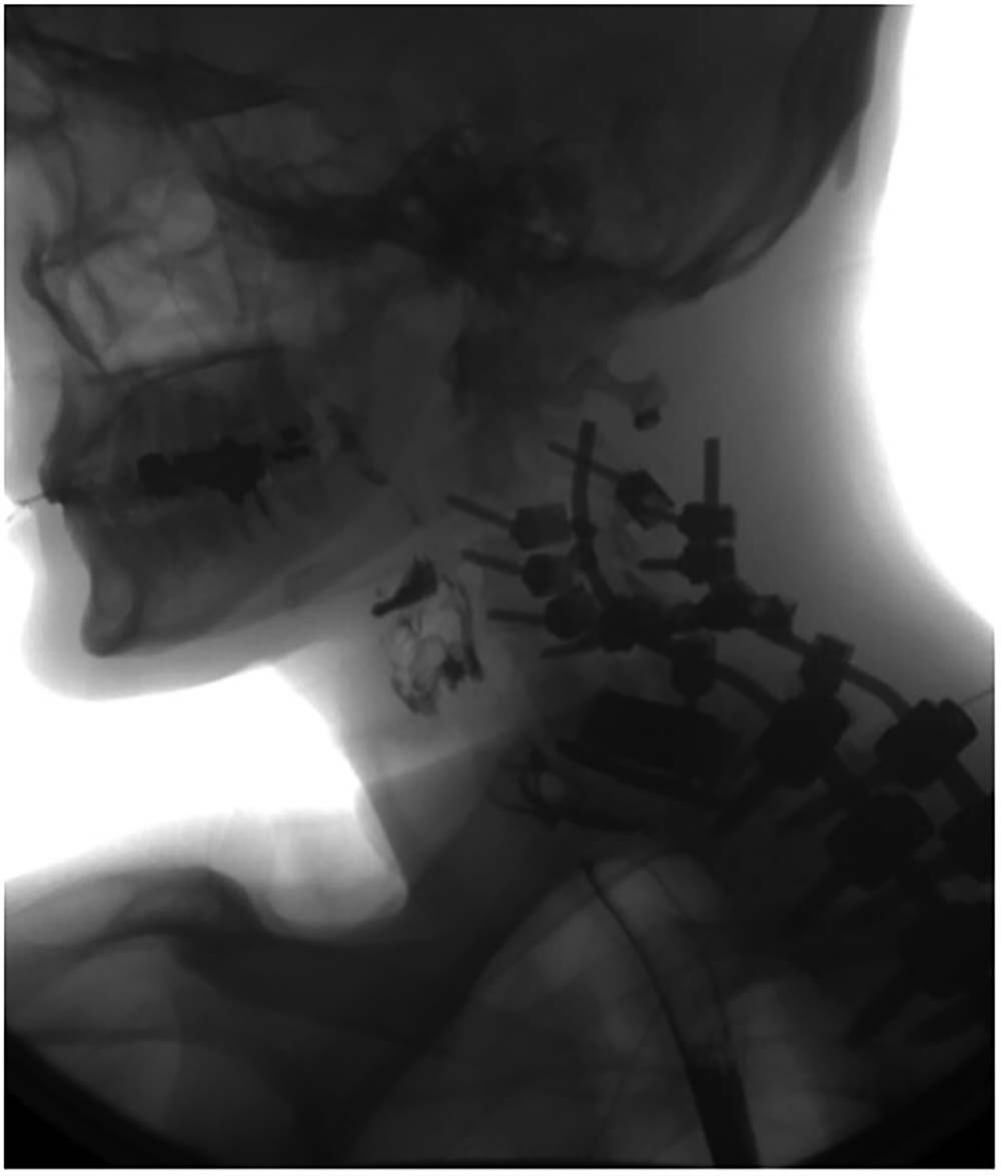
Swallow study demonstrating extraluminal leakage of contrast posteriorly at the C6 level consistent with CT findings.
